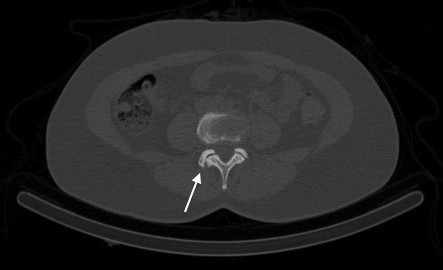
CT image from SPECT/CT demonstrating mild degenerative changes at right L3/L4 facet joint (white arrow).
